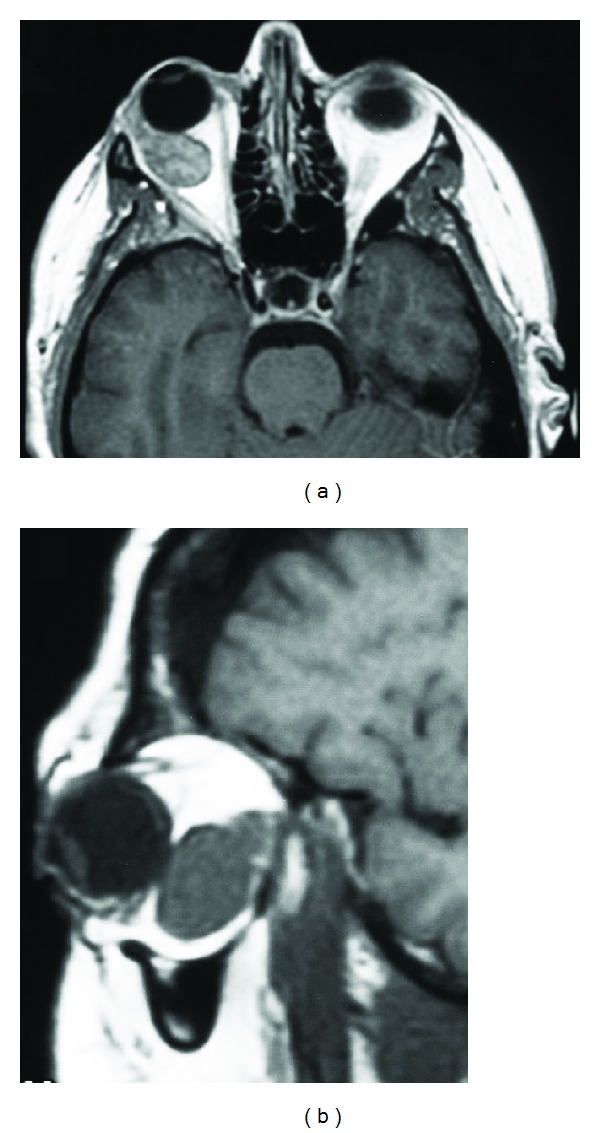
Magnetic resonance imaging of a 49-year-old female 6 weeks prior to removal of a right sided 22.9 mm cavernous hemangioma.
